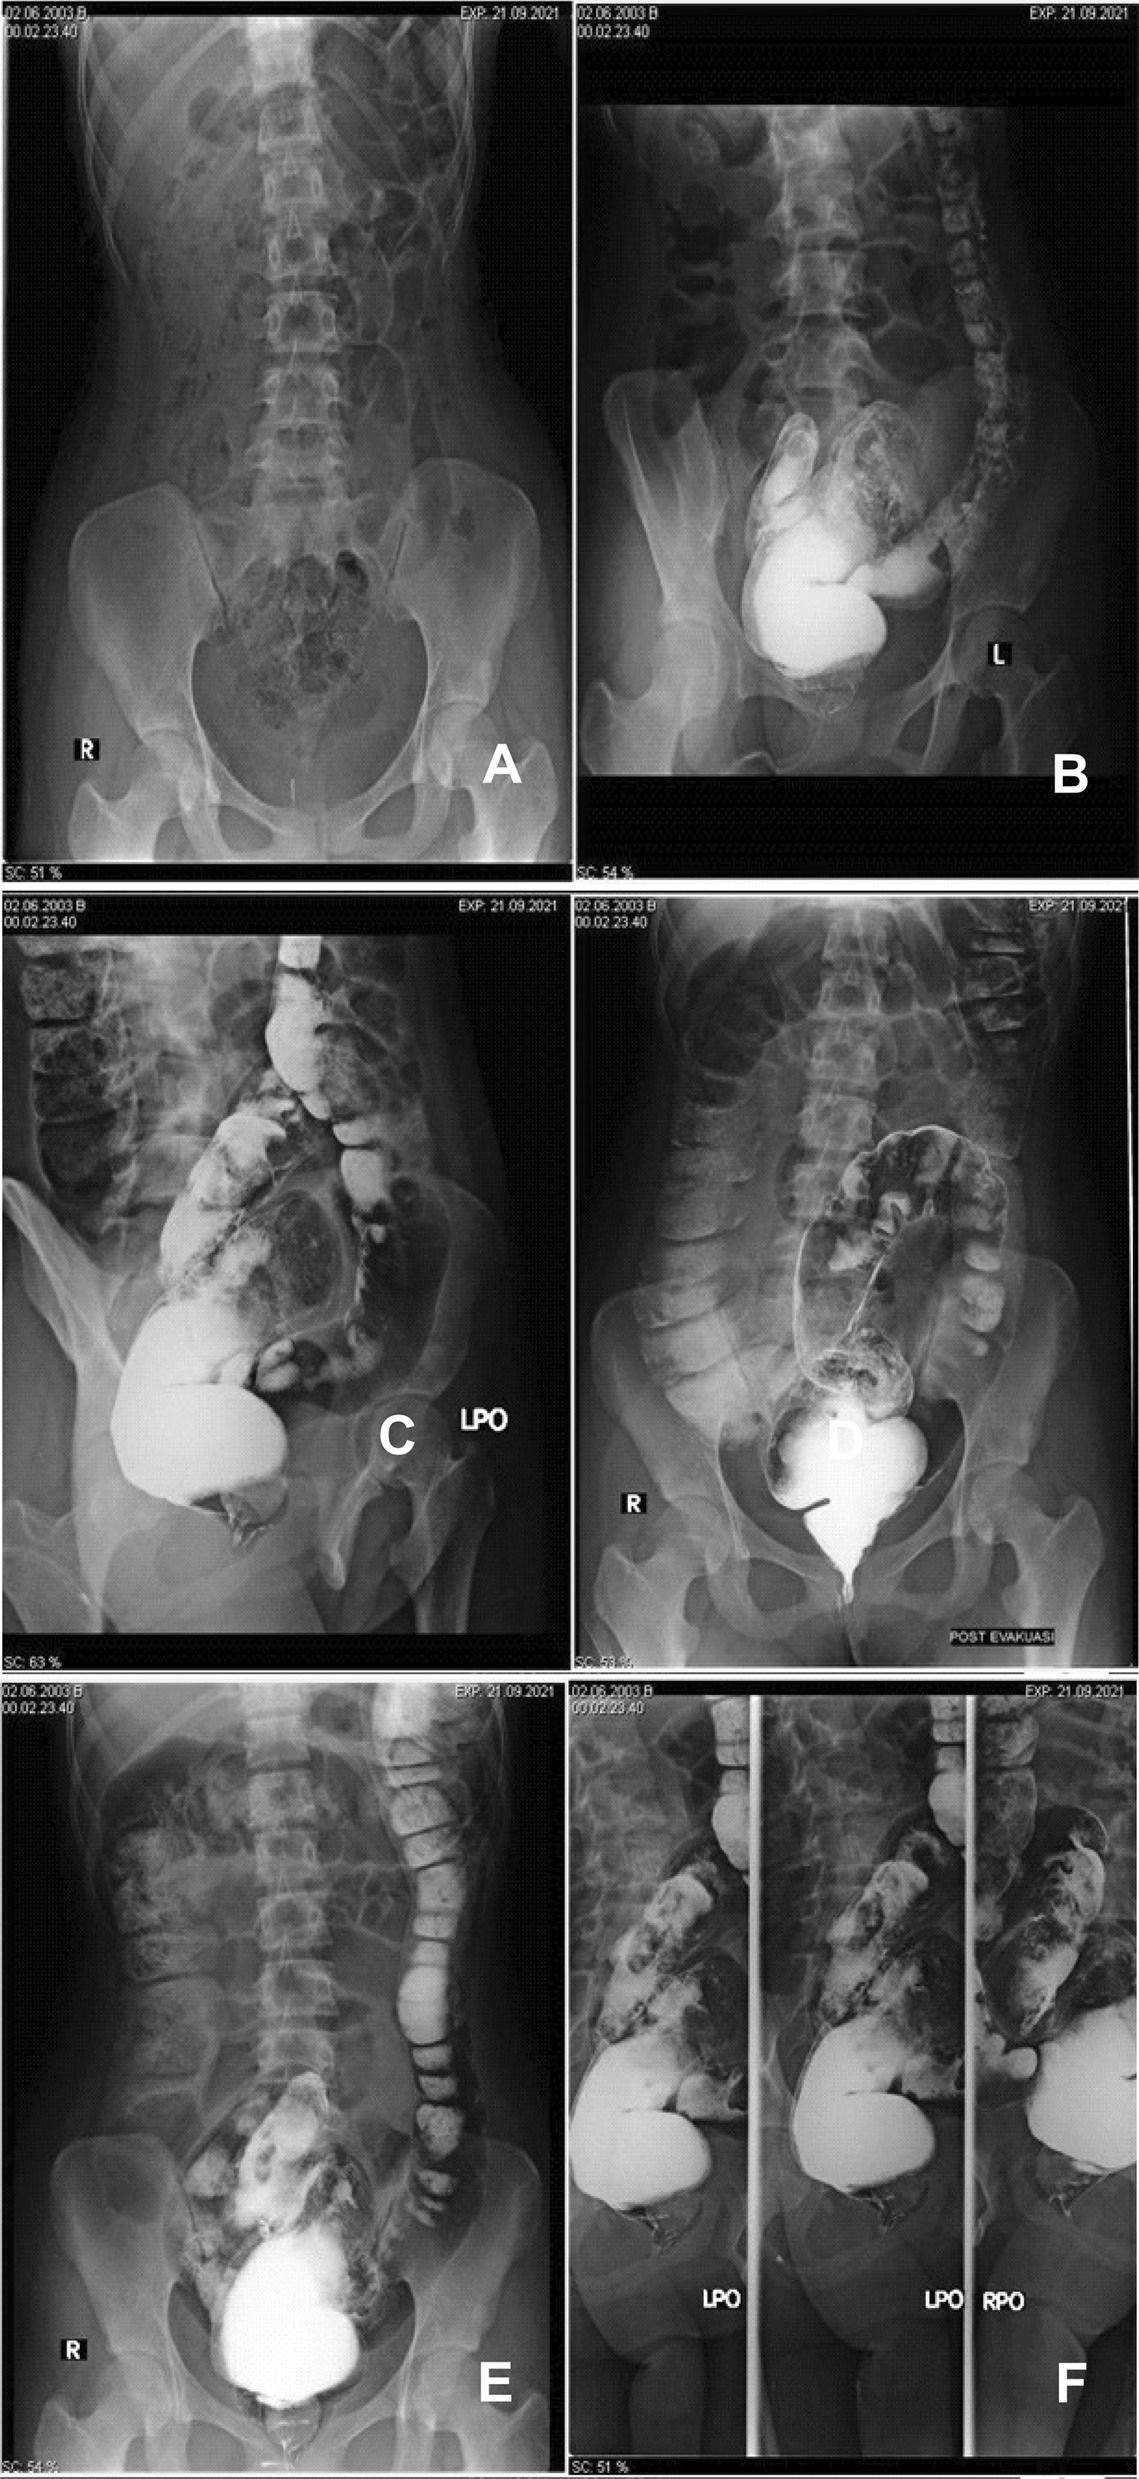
A Abdominal plain radiograph. This figure revealed that the air distribution in the colon and small intestine is increased with coiled spring appearance. There was neither stepladder appearance nor subdiaphragmatic free air. B–F Contrast enema study radiograph. The study showed that the sigmoid colon was dilated and the rectum was narrowed, with rectosigmoid index less than 1 and irregular rectosigmoid mucosa
